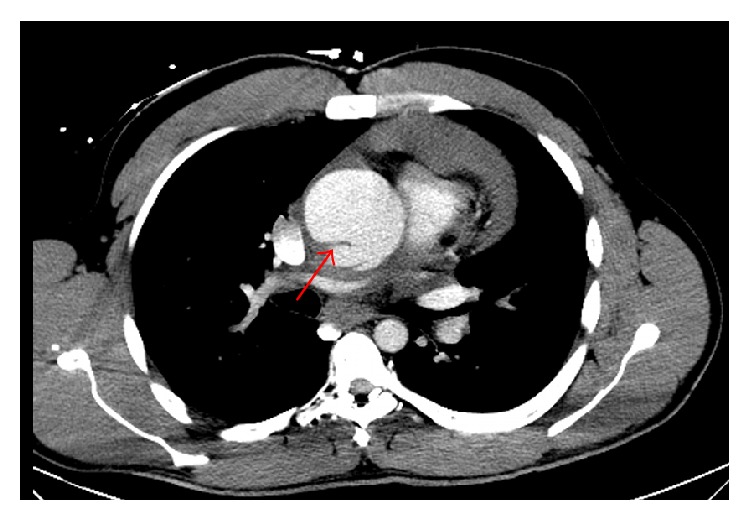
CT scan of chest with contrast showing dissection flap in ascending aorta (red arrow).
